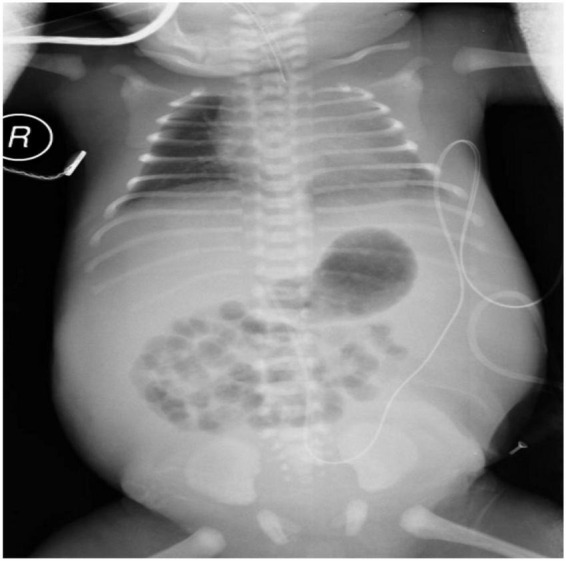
Radiograph of the neonate’s chest and abdomen showing generalized edema and tense distended abdomen with a mount of ascites.
